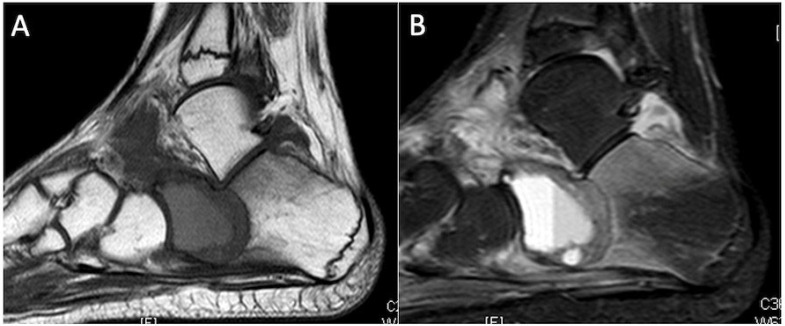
T1-weighted (A) and STIR (B) sagittal MRI demonstrating a chondroblastoma of the anterior calcaneus, with florid perilesional intramedullary oedema, greater in volume than the lesion itself.
